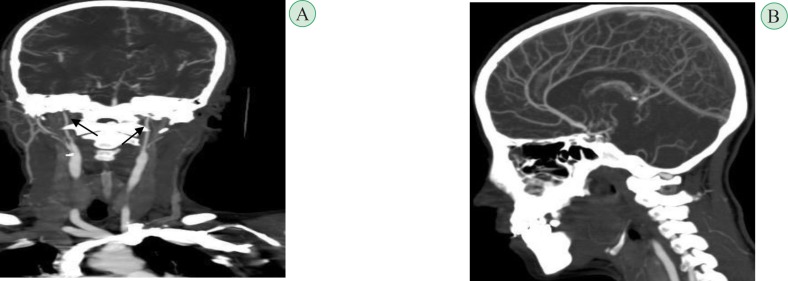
Brain and cervical CT-Angiography. A-Note bilateral narrowed internal carotids (arrows). B-collateral vascular networks appear "puff of smoke" pattern
